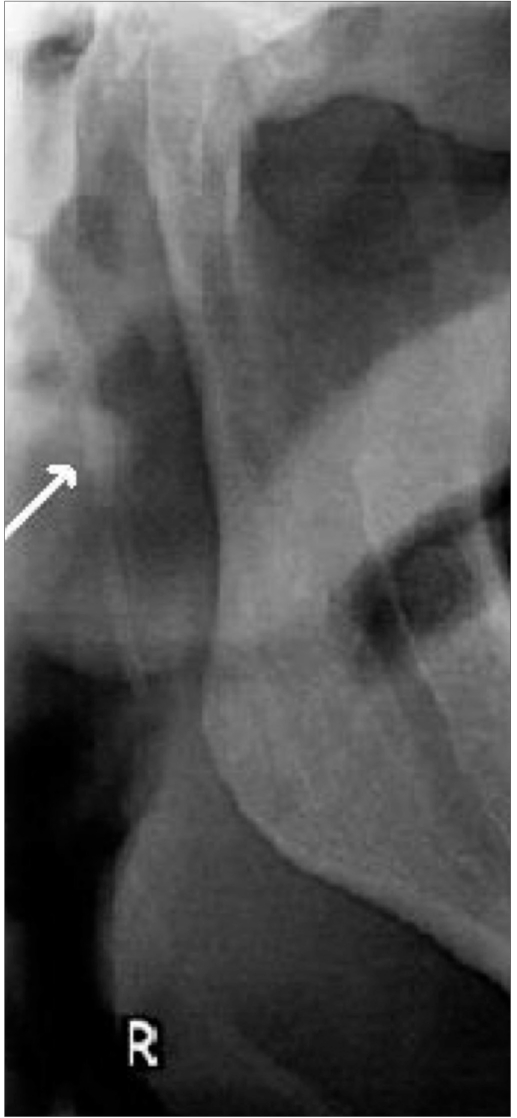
The orthopantomogram shows a partially calcified styloid process (arrow)
